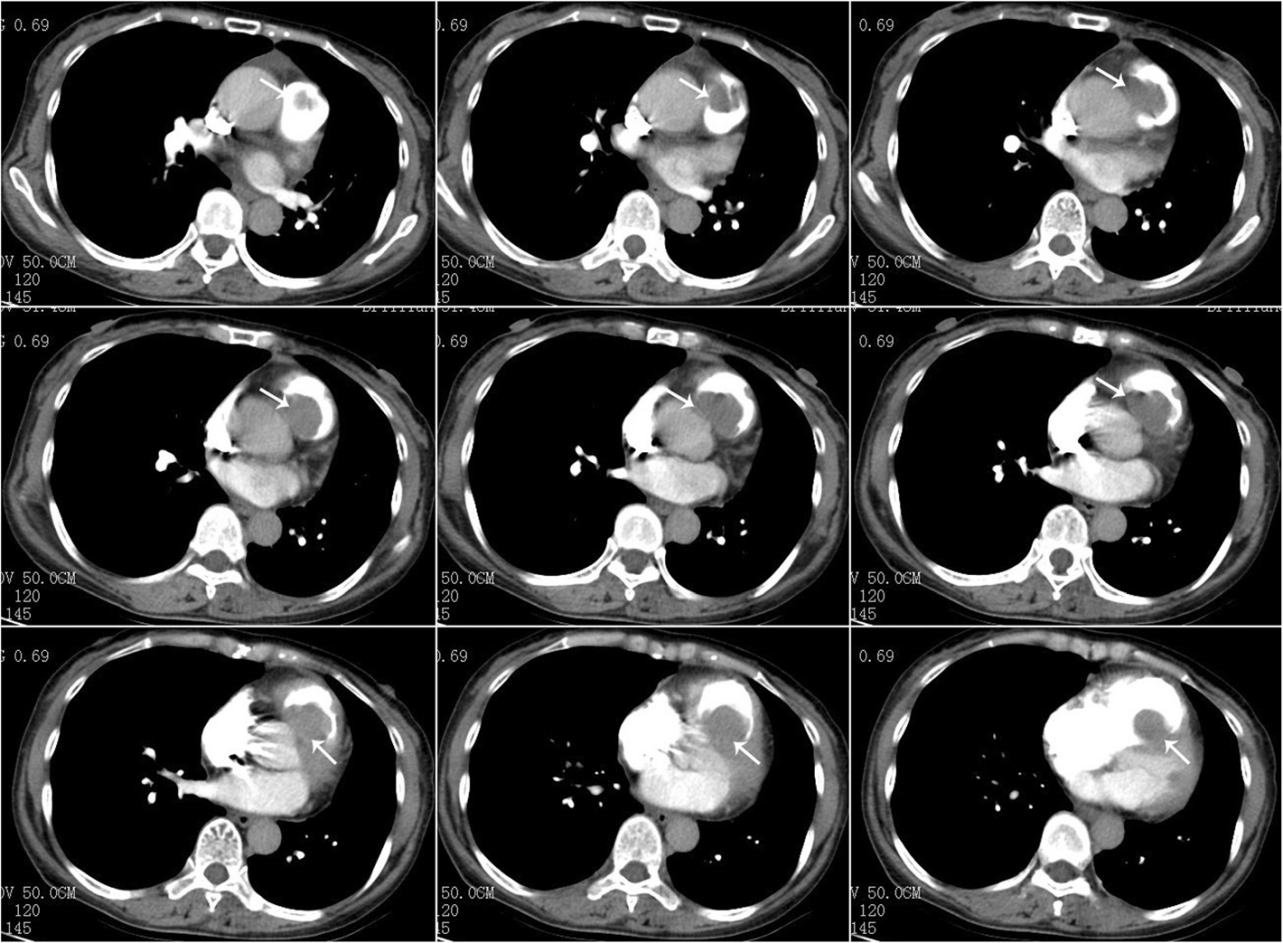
Preoperative, contrast-enhanced, computed tomographic scan of the chest. A large mass measuring 5cm × 6cm, filling defects in the main pulmonary artery and right ventricle and hydropericardium can be seen (arrows).
